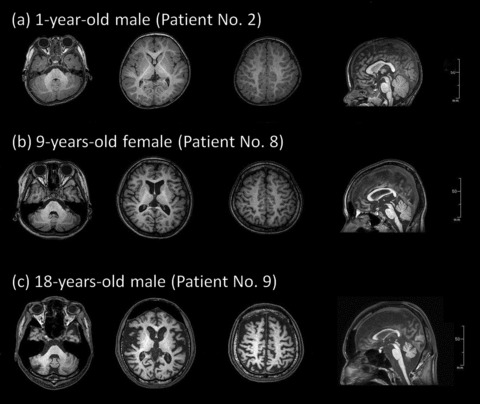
Three axial views and one sagittal view from T1-weighted MRI images in (A) 1-year-old, (B) 9-year-old, and (C) 18-year-old XPA patients.
